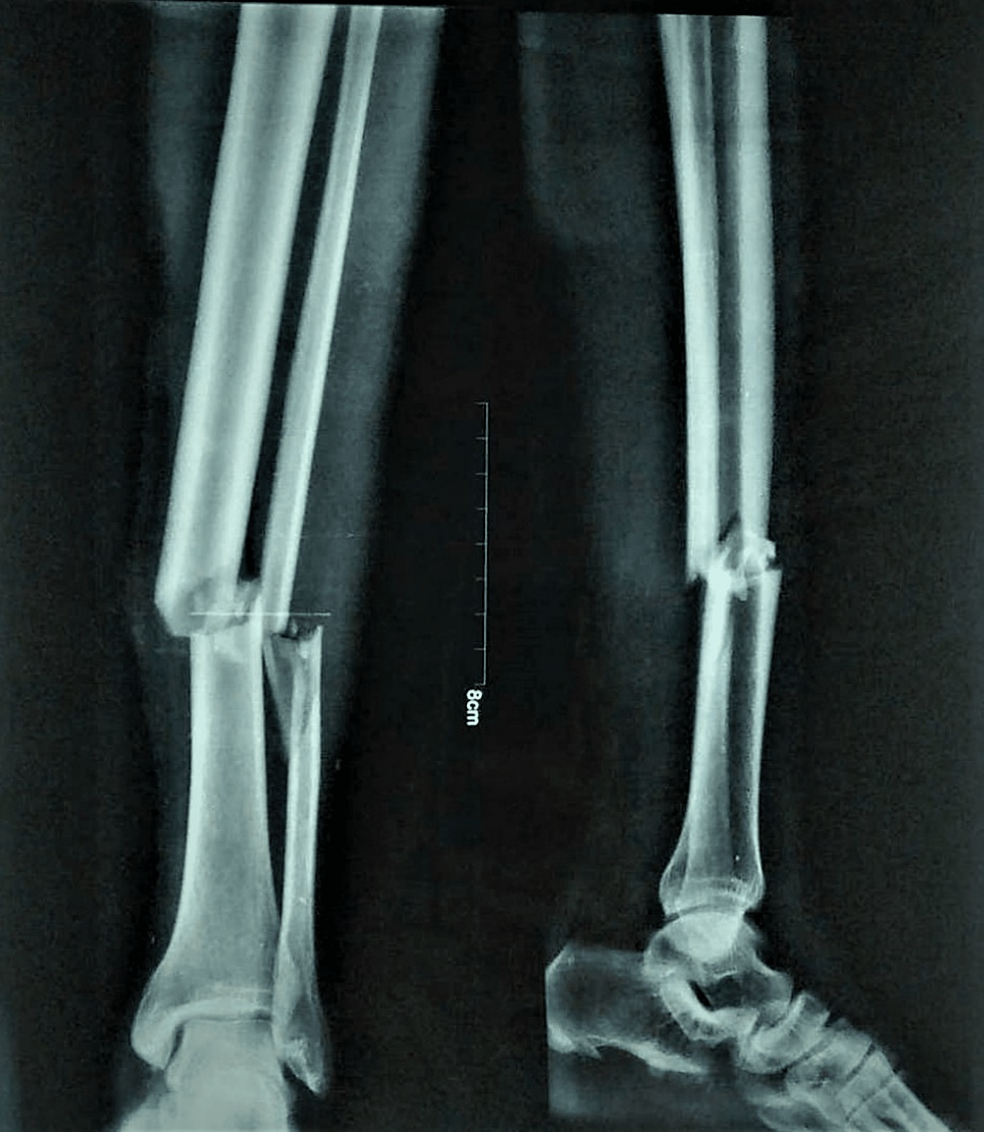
Preoperative X-ray (anteroposterior and lateral views)
